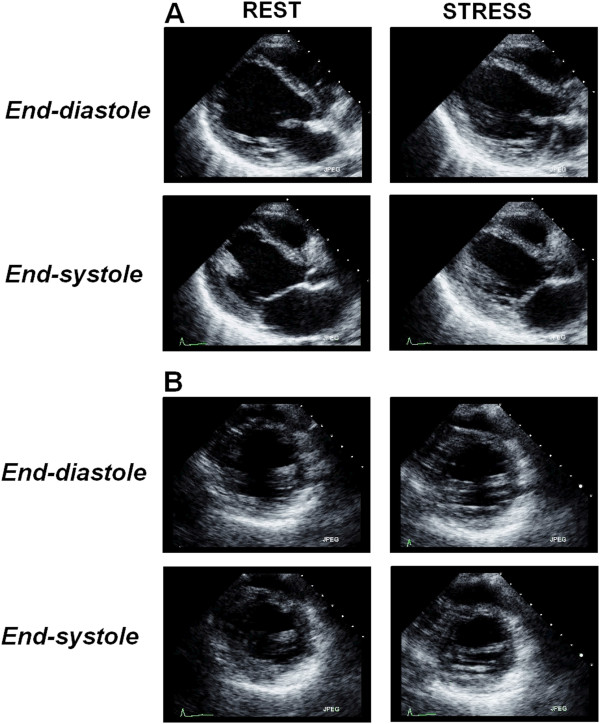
Donor #2 in Table 1. An example of a stress echocardiogram in a brain-dead potential donor, showing end-diastolic and end-systolic frames at rest and following stress using Dipyridamole (0.84 mg/kg in 6’) [see Additional file 1]. Left ventricular interventricular septum and apical wall a-kinesia are shown in parasternal long-axis (panel A) and short-axis (panel B) chamber views. At peak stress an incomplete viability response is shown. The donor was considered unsuitable for heart donation.
